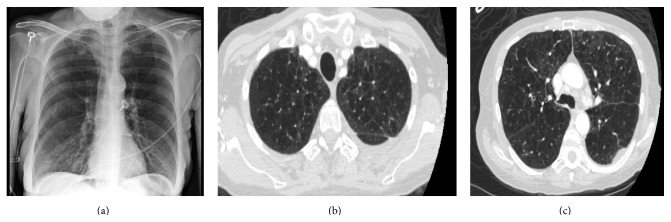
Chest X-ray and chest CT findings in Case 1. Chest X-ray findings on day of admission demonstrating hyperinflated lung fields. Chest CT findings 10 months before case presentation demonstrating severe centrilobular emphysema.
